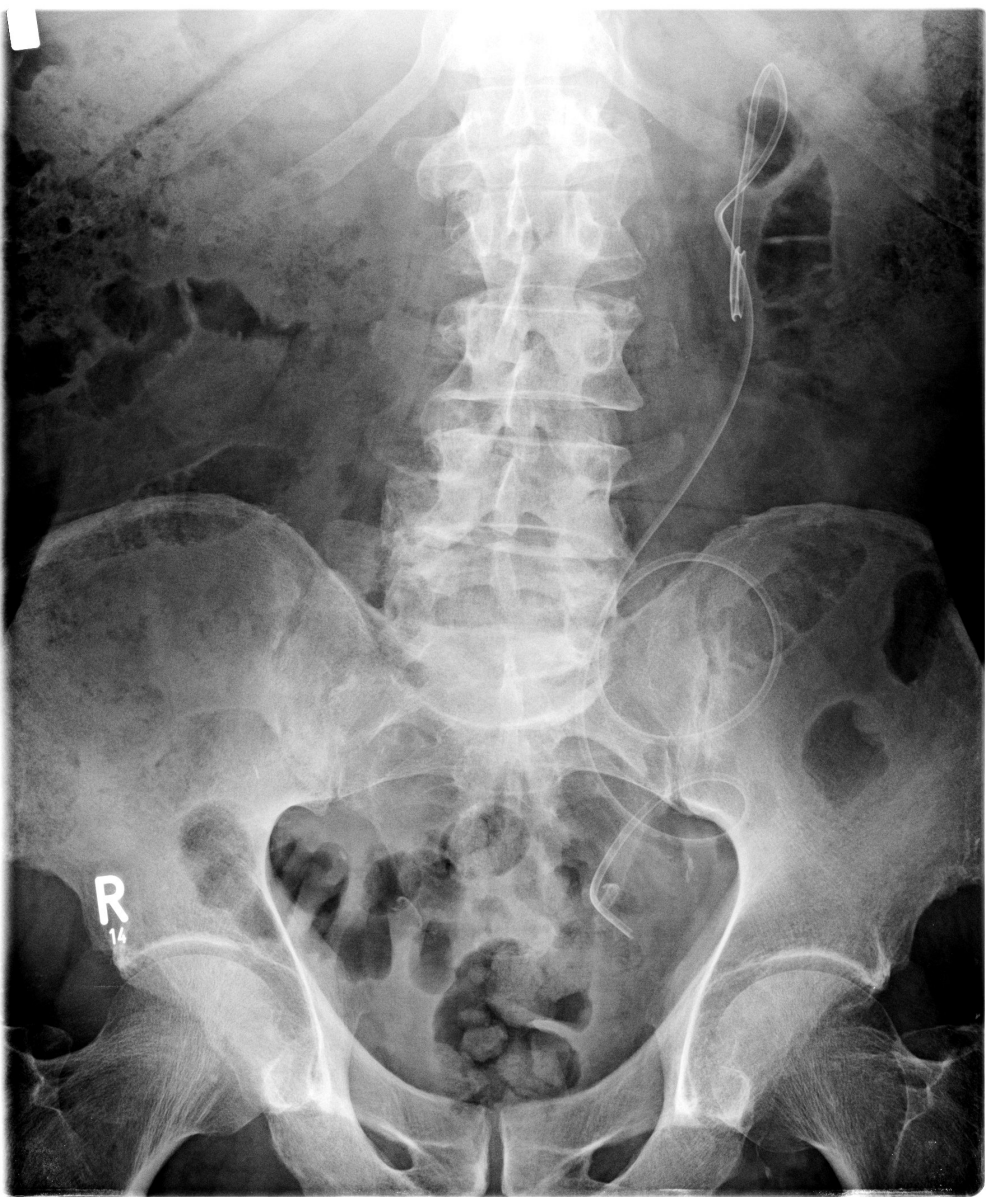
Radiograph after laparoscopic operation.
